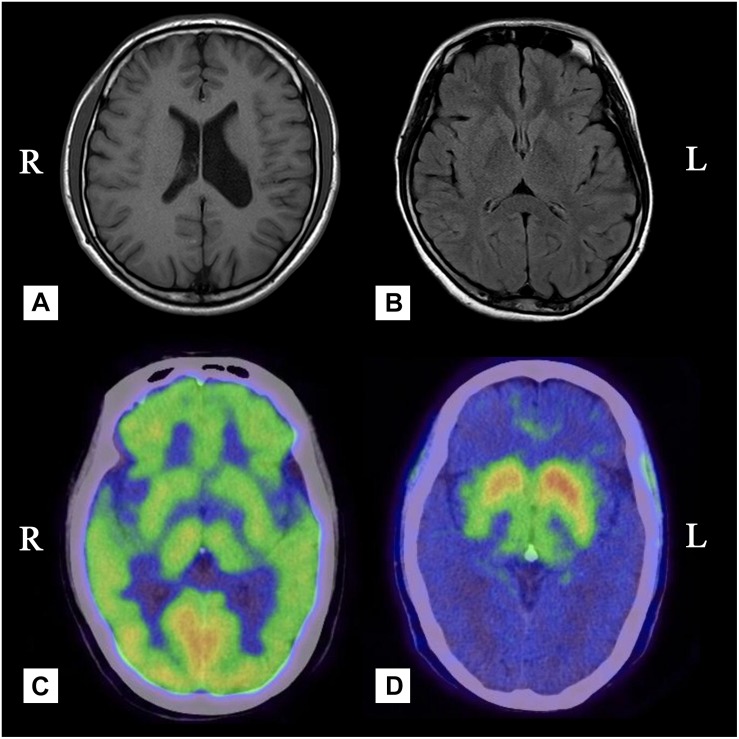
(A) Case 4, Brain MRI showing dilatation of the left lateral ventricle. (B) Case 2, Brain MRI showing mild brain atrophy but no signs of hemisphere hypoplasia. (C) Case 3, PET with [18F]-FDG was bilaterally uniform and symmetrical. (D) Case 3, there was a significant reduction in the uptake of 18F-DOPA in the contralateral posterior striatum.
